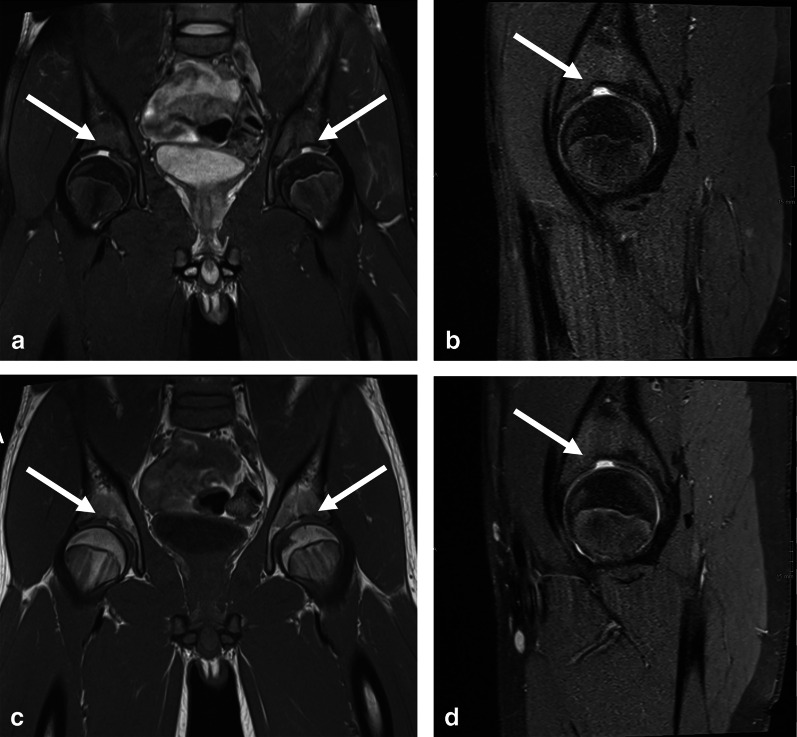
Hip-MRI of a 15-years-old, male participant without contrast with bilateral SAF type 1 (white arrow): Proton density weighted (PD) coronal both sides (a), PD sagittal right side (b), T1 weighted coronal both sides (c) and PD weighted sagittal left side (d)
